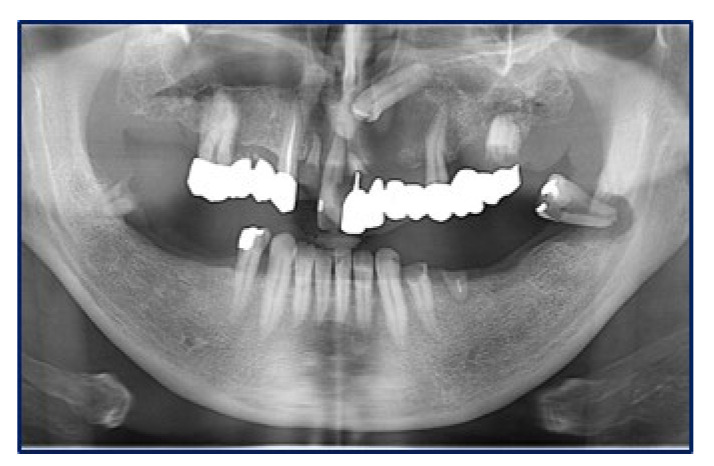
Panoramic radiogram showing an osteolytic lesion of the left anterior maxilla with undefined borders, involving the periodontal ligament of 2.1 and 2.4 as well as the retained 2.3 tooth.
